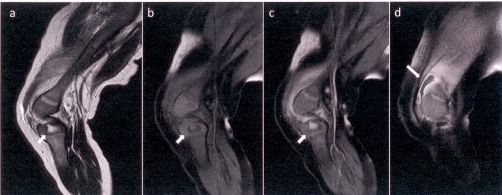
MRI study (sagittal projections). (a) T2-Weighted Images (WI). On the epiphysis of left tibia an oval area (arrow) of high signal intensity is demonstrated. (b) T1-WI with Fat Saturation demonstrates the same area (arrow) with intermediate to low signal intensity. (c) T1-WI with Fat Saturation (FS) after intravenous administration (IV) of paramagnetic substance. The lesion (arrow) avidly enhances. There is also soft tissue enhancement around the distal part of femur. (d) T1-WI with FS after IV of paramagnetic substance. Fluid collection with peripheral enhancement (arrow) in the suprapatellar pouch represents an abscess.
